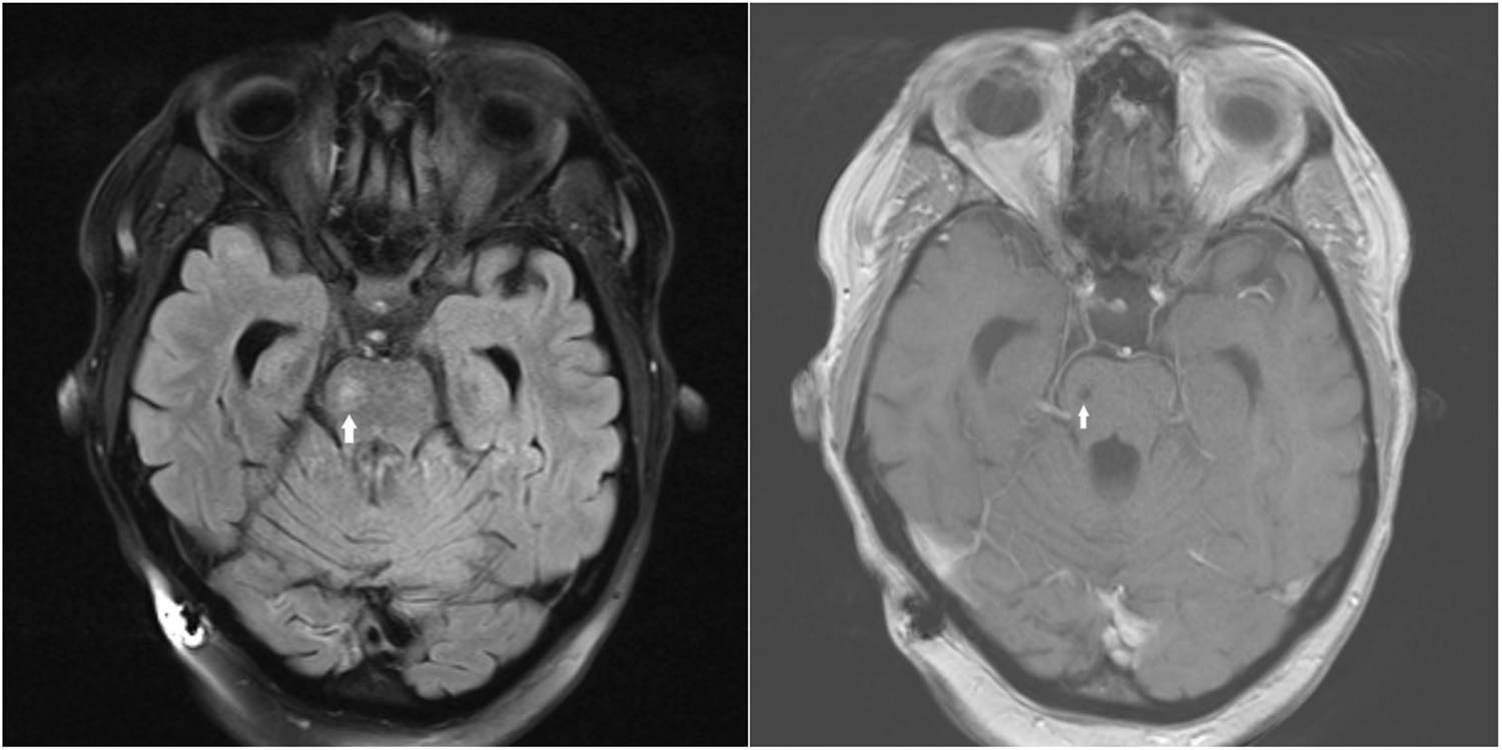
MRI revealed lacunar stroke in the right side of the pons and diffuse T2 hyperintensity and T1 hypointensity widespread lesions in the white matter of the brain (white arrows)
